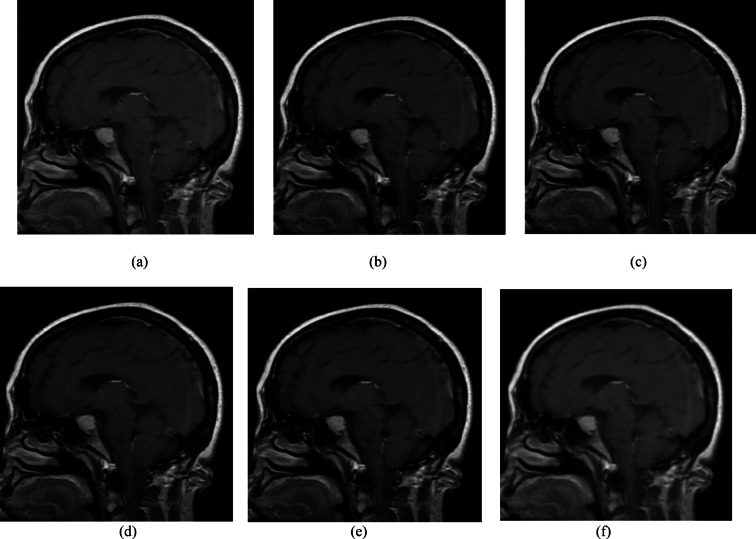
Visual comparison of MRI images under different denoising methods. (a) Original noisy image, denoised image using(b) Gaussian Pyramid, (c) wavelet-Haar, (d) wavelet-coif4, (e) wavelet-db4, and (f) wavelet-sym4.
